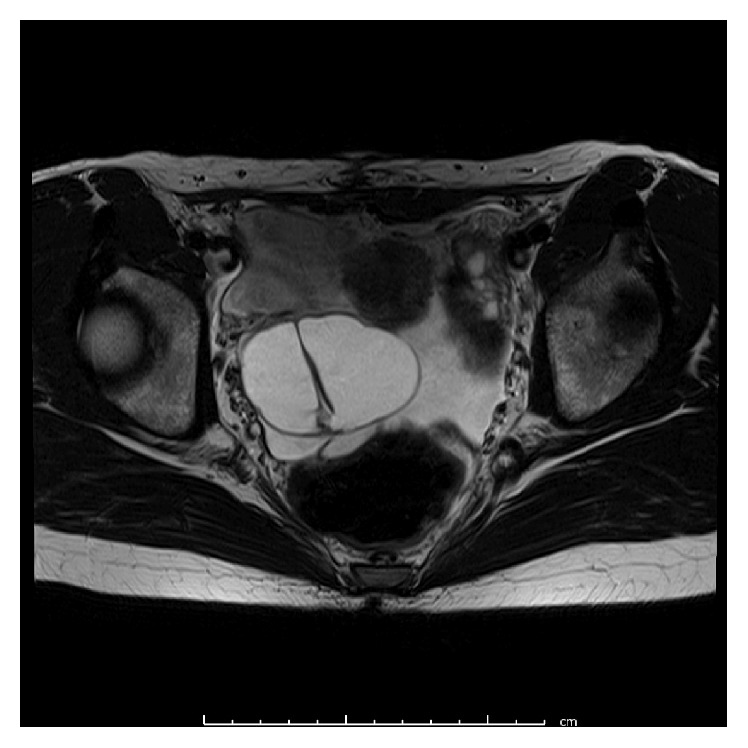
Pelvic magnetic resonance imaging (T2-weighted image) before the third surgery of Case  2. Right ovarian tumors are detected (multiple cystic, 6.3 × 4.9 cm in diameter).
